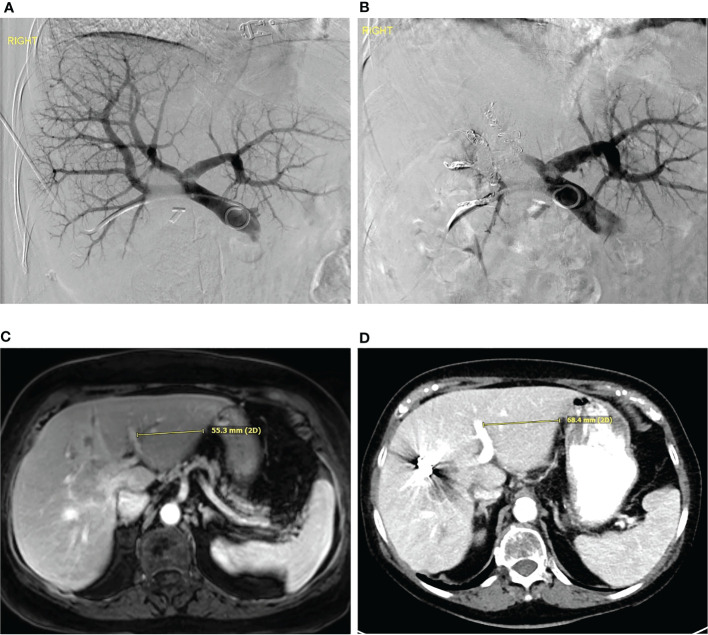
Portal vein embolization (A) Digitally subtracted percutaneous transhepatic portovenogram demonstrates patent main, left and right portal veins. The portal vein was embolized with particles followed by coil embolization. (B) Digitally subtracted portovenogram after portal vein embolization demonstrates flow only to the left hepatic lobe. (C) Pre-procedure MRI and (D) post portal vein embolization CT demonstrates hypertrophy of the left hepatic lobe.
